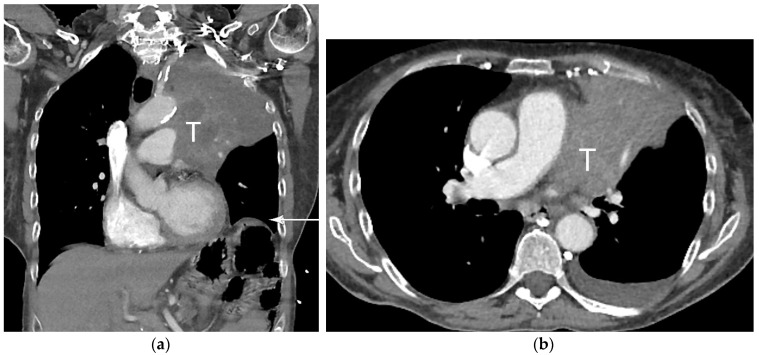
T4 disease. (a) Axial contrast-enhanced CT, and (b) coronal CT show 10 cm left upper lobe primary tumor (T) invading the mediastinum. Involvement of the left phrenic nerve resulted in elevation of the left hemidiaphragm (arrow). Involvement of the phrenic nerve is T3 disease. However, both size of greater than 7 cm and mediastinal invasion are T4 descriptors, dictating the T classification in this case.
